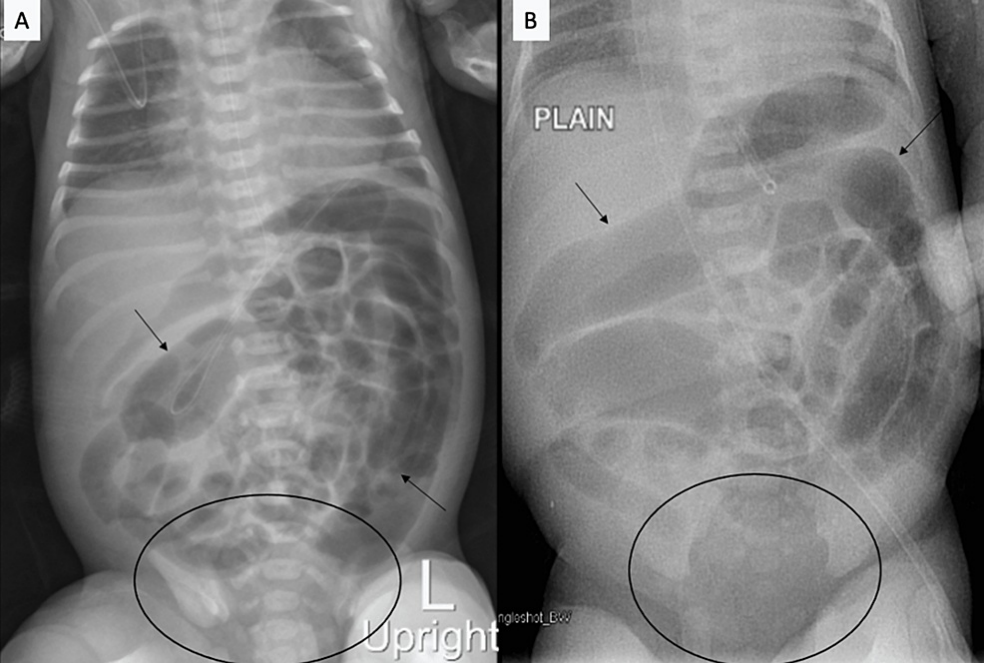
Abdominal radiographs of the neonate in upright (A) and supine (B) positions: showed gas-filled dilated bowel loops in the abdominal cavity (black arrow) with a paucity of rectal gas (black circle). No free peritoneal air or calcification was seen. These findings suggest the diagnosis of low-type intestinal obstruction with a differential of Hirschsprung disease at the top of the list.
